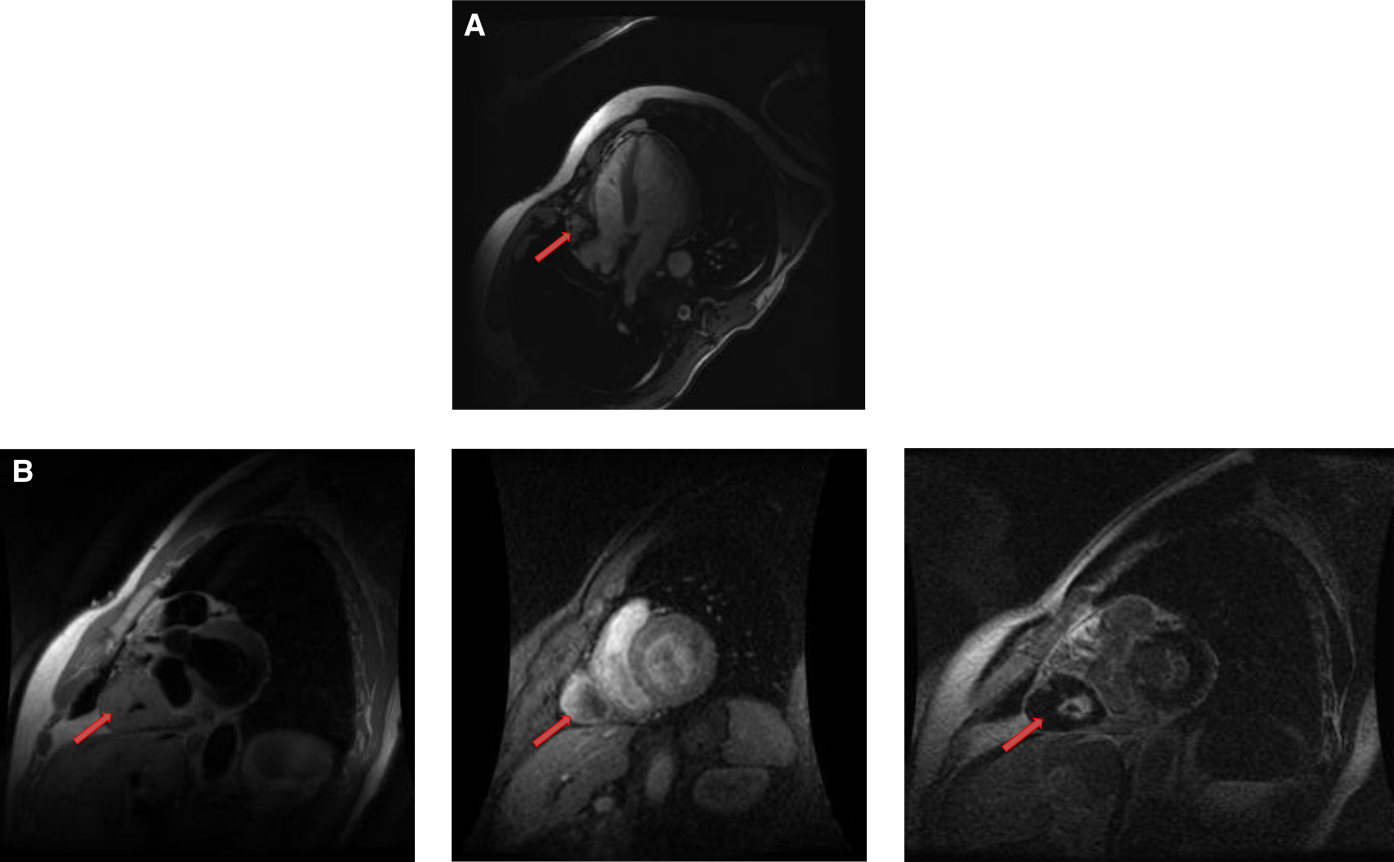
A mature teratoma in a 16 years old female patient. (A). Contrast enhancing CT chest showing a calcified, fatty lesion inside heart, calcifications are multifocal representing huge mature cardiac teratoma.
